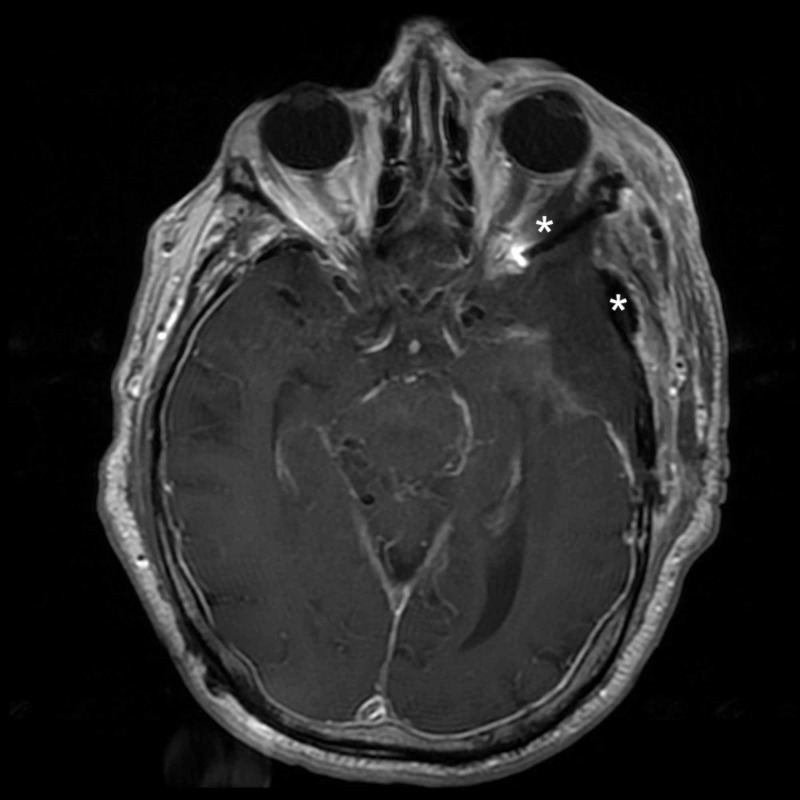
Postoperative axial MRI demonstrating resection of the intraorbital and intramuscular tumor component * = Areas of prior tumor invasion of the lateral orbit and temporalis muscle MRI: magnetic resonance imaging
